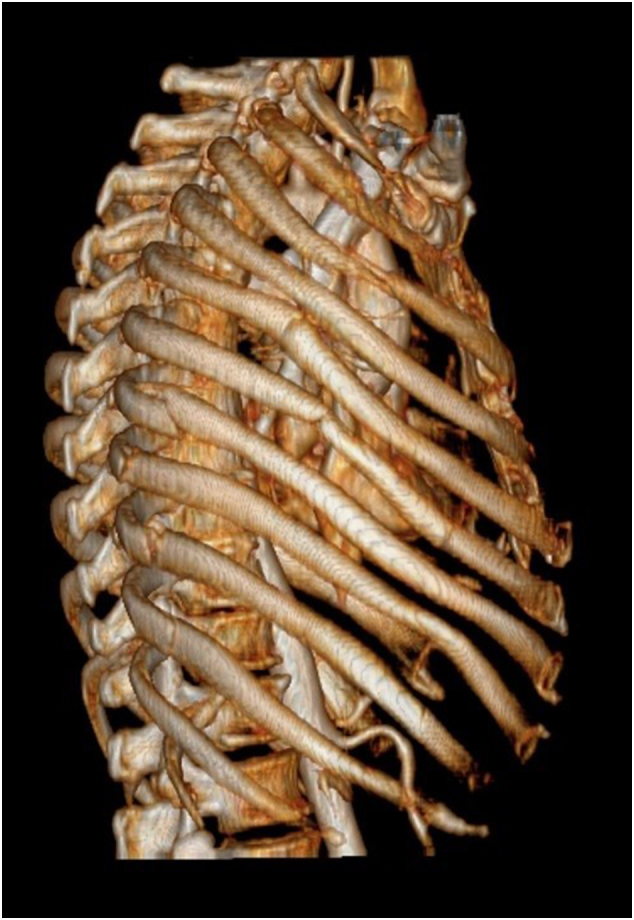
3D Computed tomography rendering demonstrating laterally displaced fractures of ribs five and six.
